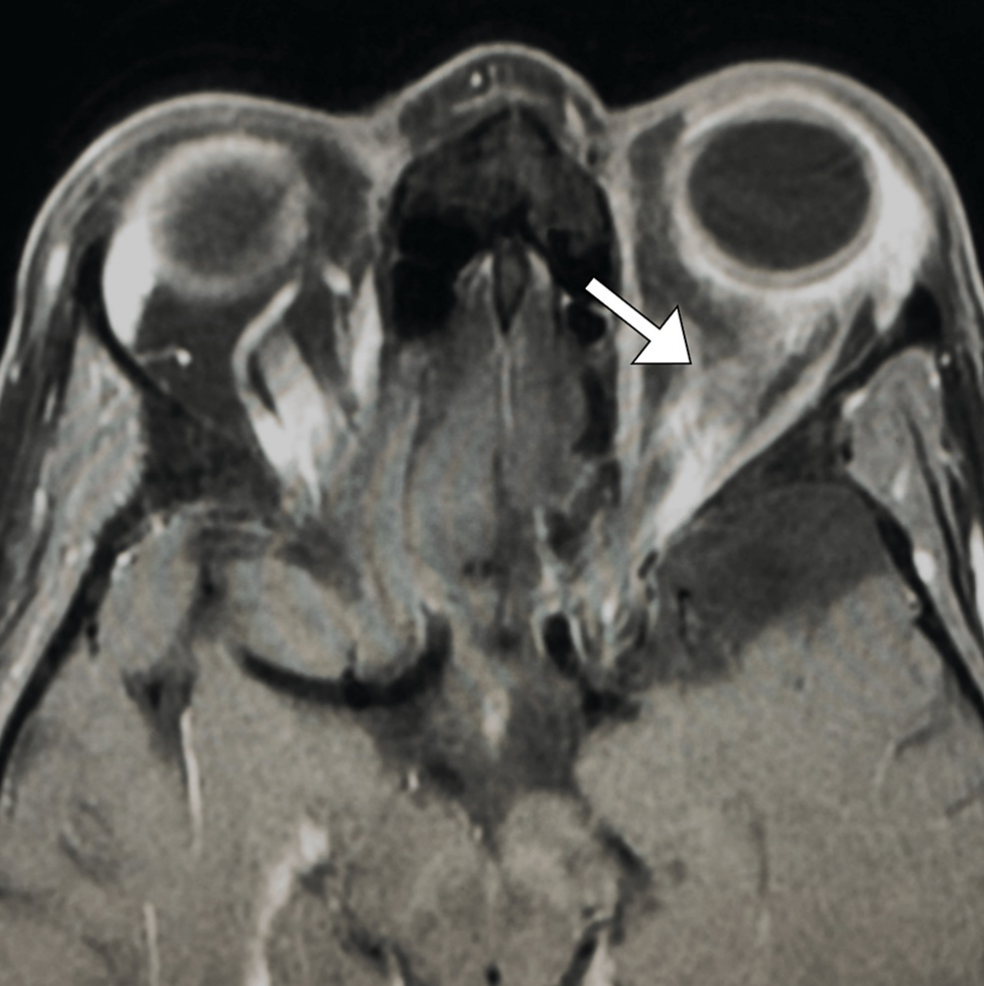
Axial MRI image with contrast revealing left retrobulbar soft tissue abnormalities, optic nerve enhancement, and mild swelling (arrow). MRI: magnetic resonance imaging
